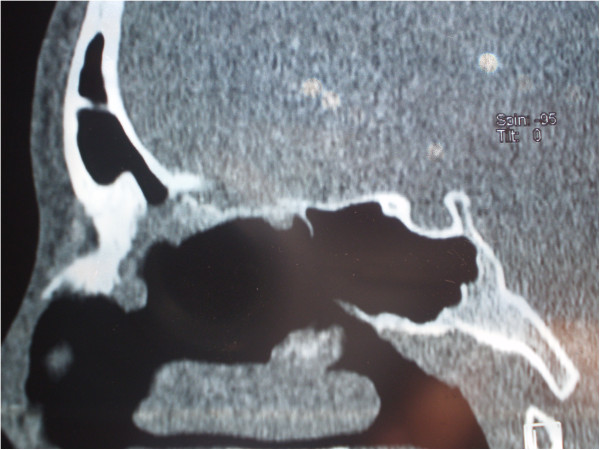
Sagittal multislice computed tomography one year postoperatively.
